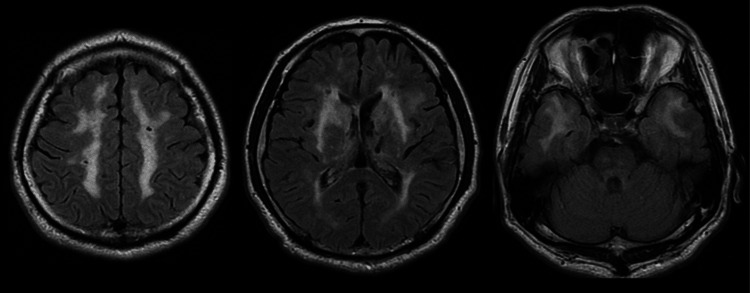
Brain magnetic resonance imaging (MRI) results five months after onset T2-weighted fluid-attenuated inversion recovery (FLAIR) brain MRI showing widespread confluent white matter hyperintensities, including involvement of the bilateral temporal tips.
